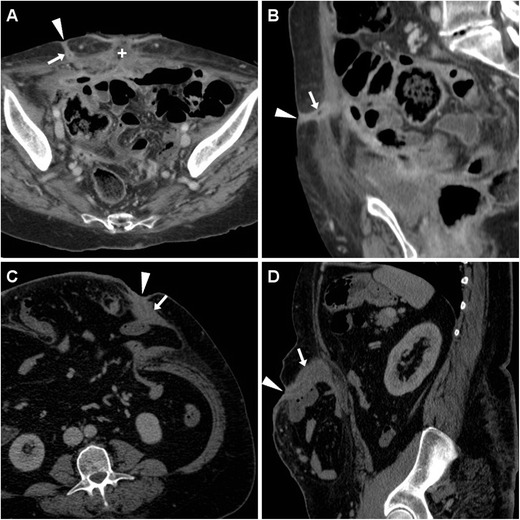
Early postoperative ECF in a 74-year-old woman following cytoreductive surgery for advanced endometrial carcinoma with peritoneal carcinomatosis. Characteristic CT (A, B) findings included enhancing “tram-track” paired structures (arrows) crossing from the peritoneal cavity through the anterior abdominal wall to the EFO (arrowheads), separated from the recent laparotomic access site (+). The ECF ultimately healed with prolonged in-hospital conservative treatment Another ECF developing in a 54-year-old obese woman with post-incisional abdominal evisceration: corresponding CT (C, D) appearance included thick-walled enhancing fistula (arrows) coursing from a herniated small bowel loop to the focally retracted EFO (arrowheads). Surgical treatment included ECF debridement and mesh hernioplasty
